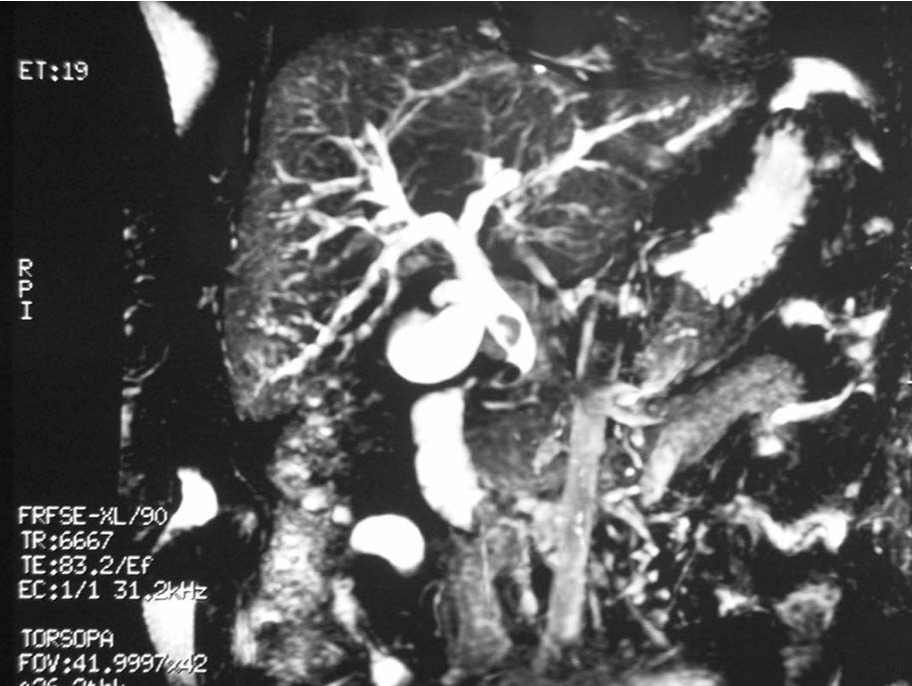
Magnetic resonance imaging. Hook-shaped appearance and CBD stones are observed.
